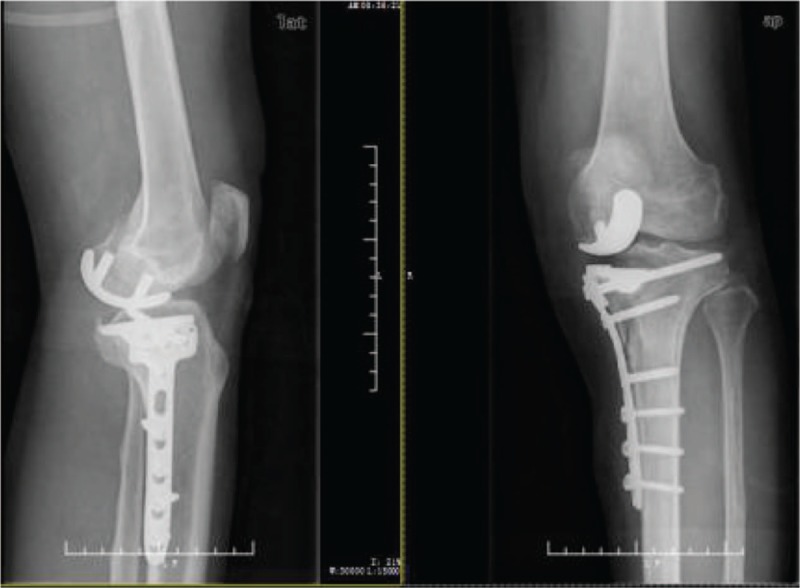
Review of X-rays on the first postoperative day. Plates and screws were well applied. The screws were in good position and did not interfere with the position of the prosthesis.
